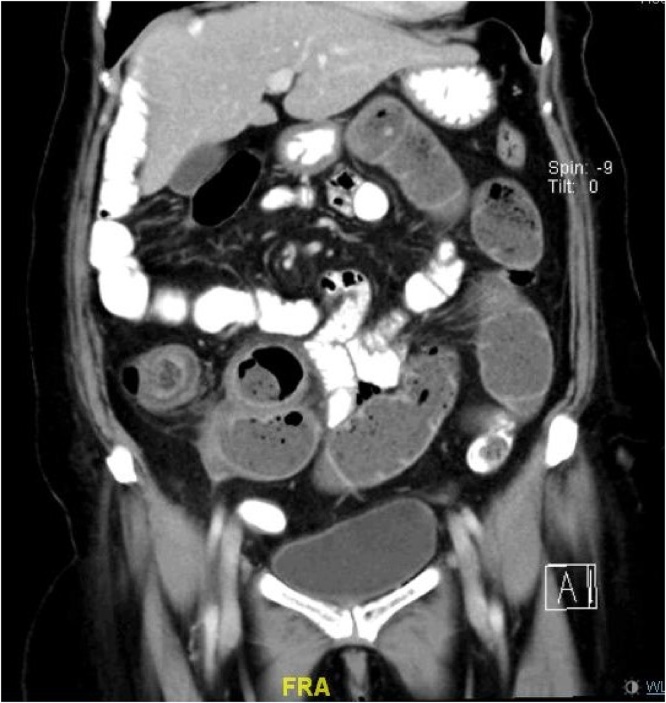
Intussusception seen on CT.
